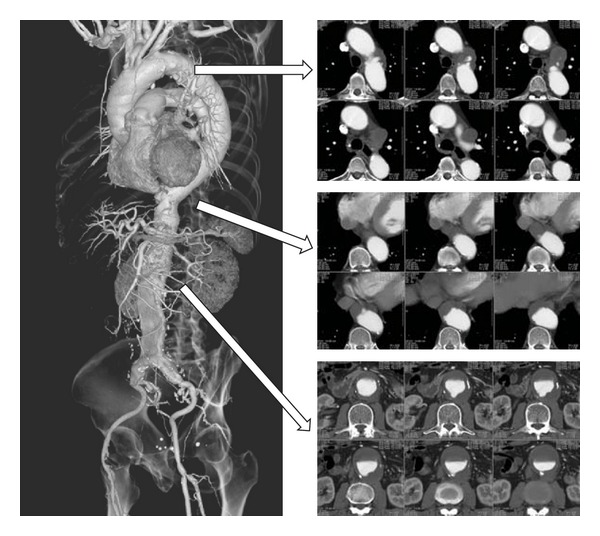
Contrast CT revealed an abdominal aortic aneurysm (anteroposterior diameter, 55 mm) with a partially protruded posterior wall, a saccular thoracic arch aneurysm, and an atheroma-rich “shaggy aorta”.
